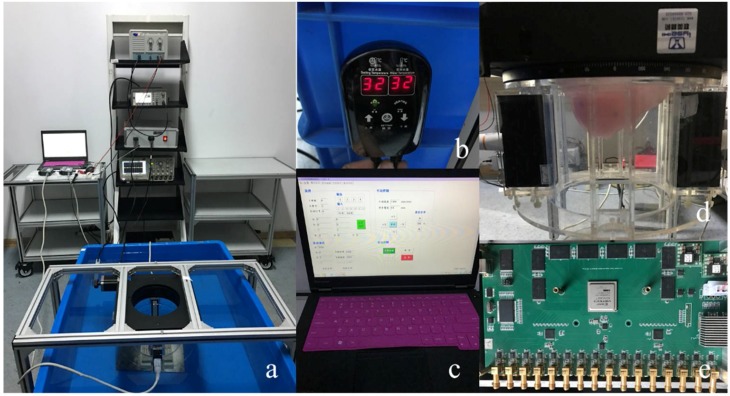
(a) Experimental platform system. Placement of breast in the ring; (b) Constant temperature heater; (c) Personal computer workstation; (d) Mechanical alignment device. Transducer ring configuration; (e) Ultrasonic signal transmitting and receiving circuits.
